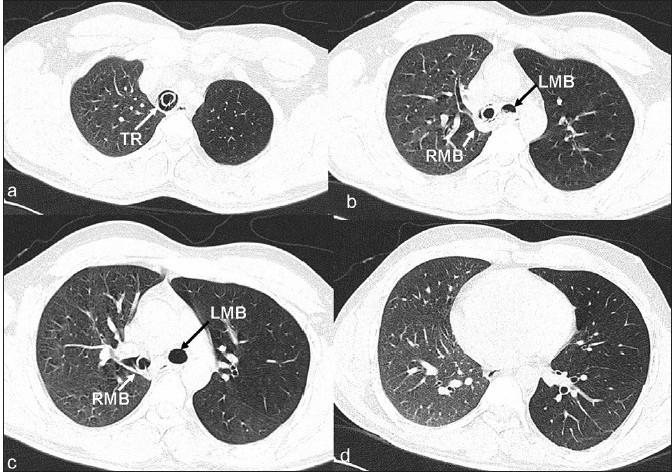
Axial sections at (a) mid thoracic tracheal, (b) carinal, (c) infra-carinal and (d) cardiac levels, respectively. Lung window images depict increased trans-radiance of the lung parenchyma with sparse vascular markings on the left side, suggestive of air trapping. The right lung shows normal CT pattern. Also note mediastinal shift to the right side. Given images also depict presence of ETT in the trachea and right main stem bronchus. TR, trachea; RMB, right main bronchus; LMB, left main bronchus
